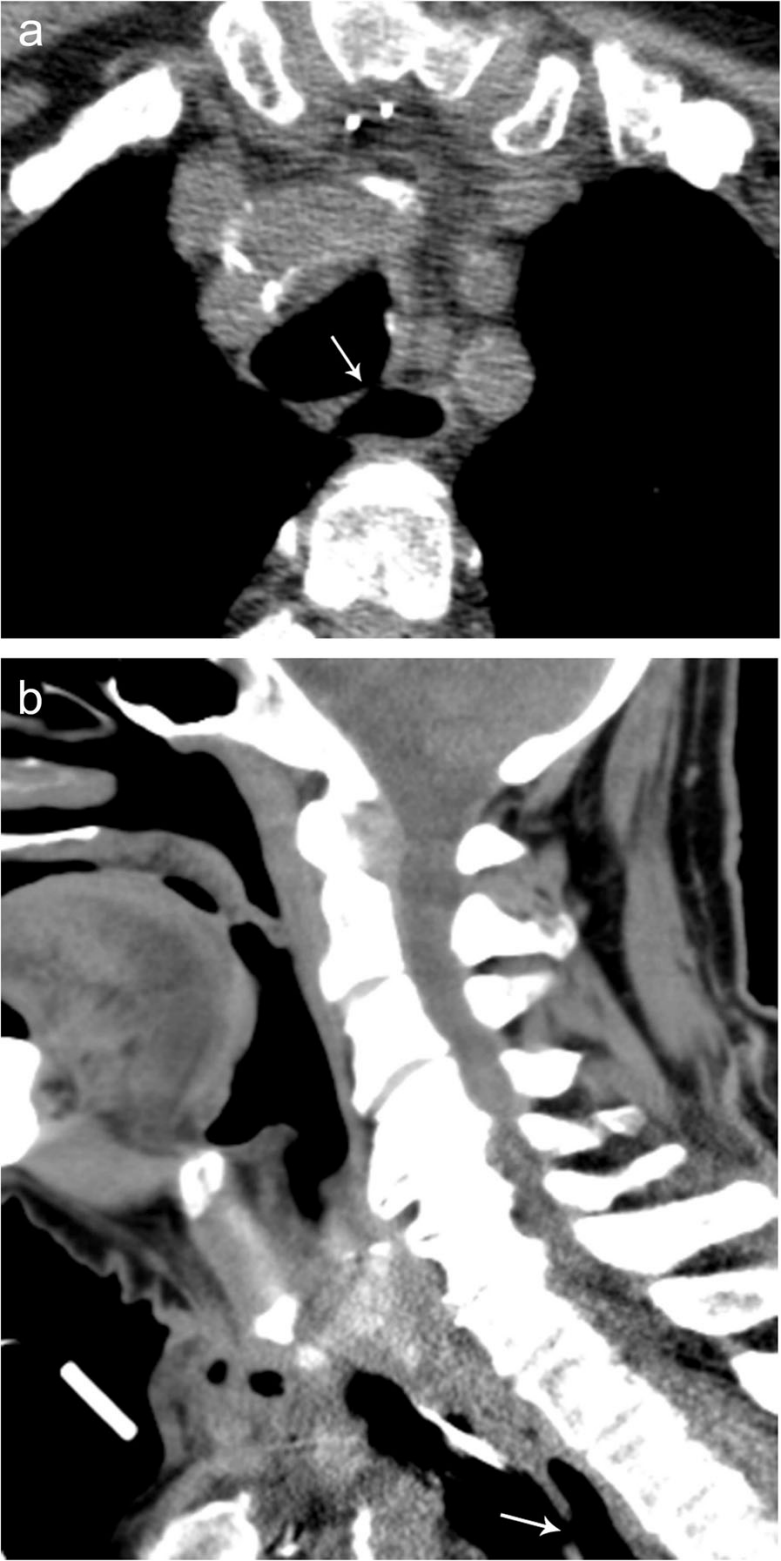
Tracheoesophageal fistula. Axial (a) and sagittal (b) noncontrast CT images demonstrating a communication (arrow) between the trachea and esophagus consistent with a tracheoesophageal fistula as a complication of esophageal stent placement in a patient with a history of esophageal trauma
